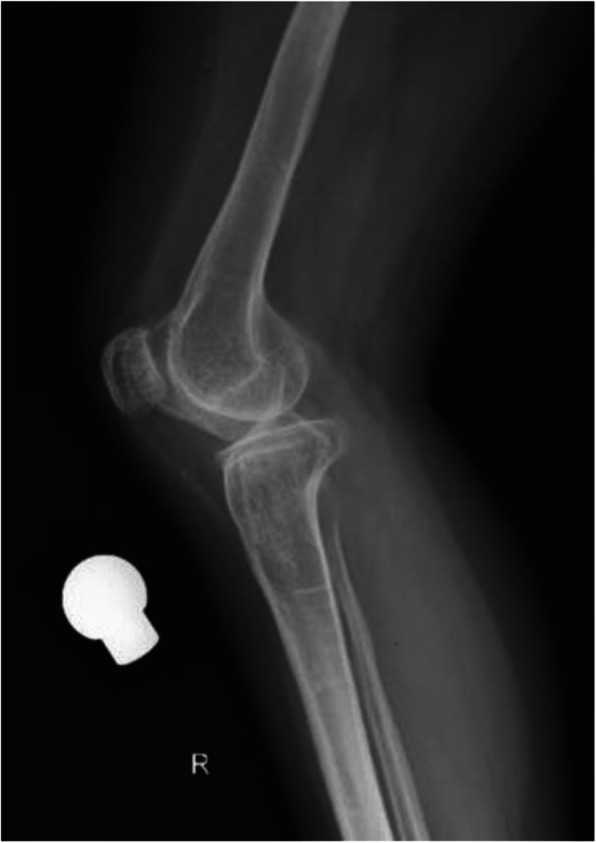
A preoperative lateral radiograph demonstrating the degenerative changes in the knee
